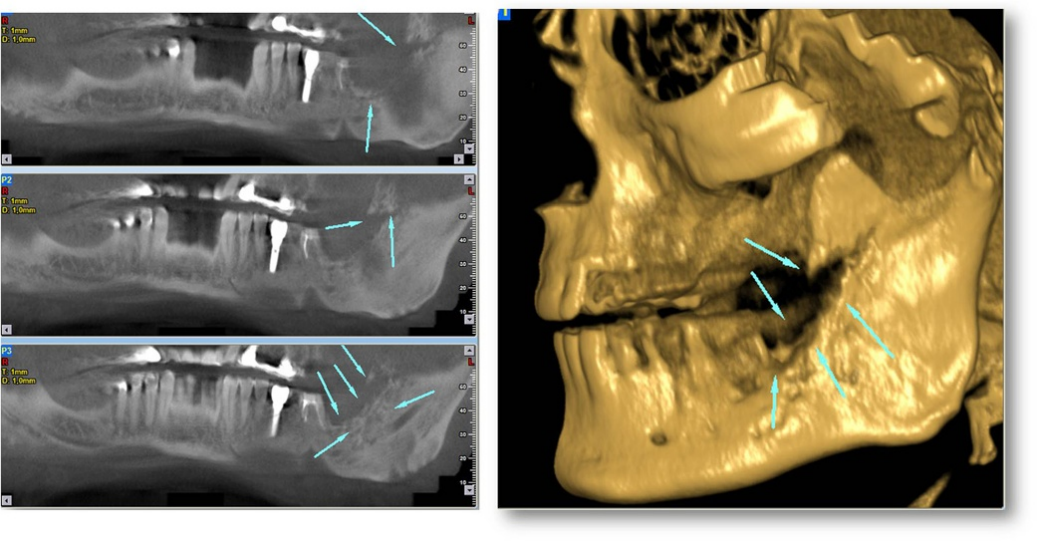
Panoramic reconstructions and 3D cone beam computed tomography (CBCT) images showing severe moth-eaten shaped erosion in relation to the mandibular nerve.
